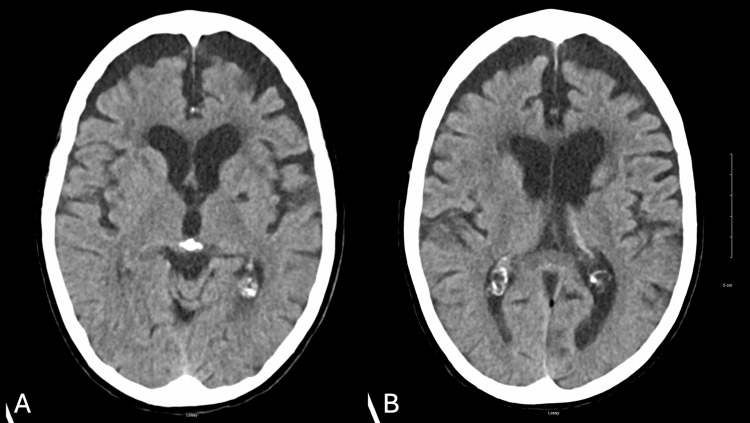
Non-contrast brain CT, axial view. CT taken during admission. No acute abnormalities noted. (A) Old lacunar infarct in left lentiform nucleus, moderate leukoecephalomalacia. (B) Asymmetric ventricles, left larger than right, moderate leukoencephalomalacia.
